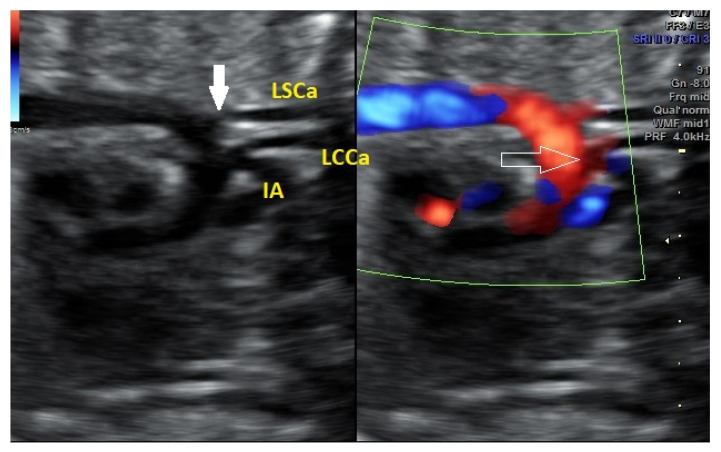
AAAV type B at 21 weeks, B mode and Doppler colour side by side. Note the common origin of IA and LCCa.
